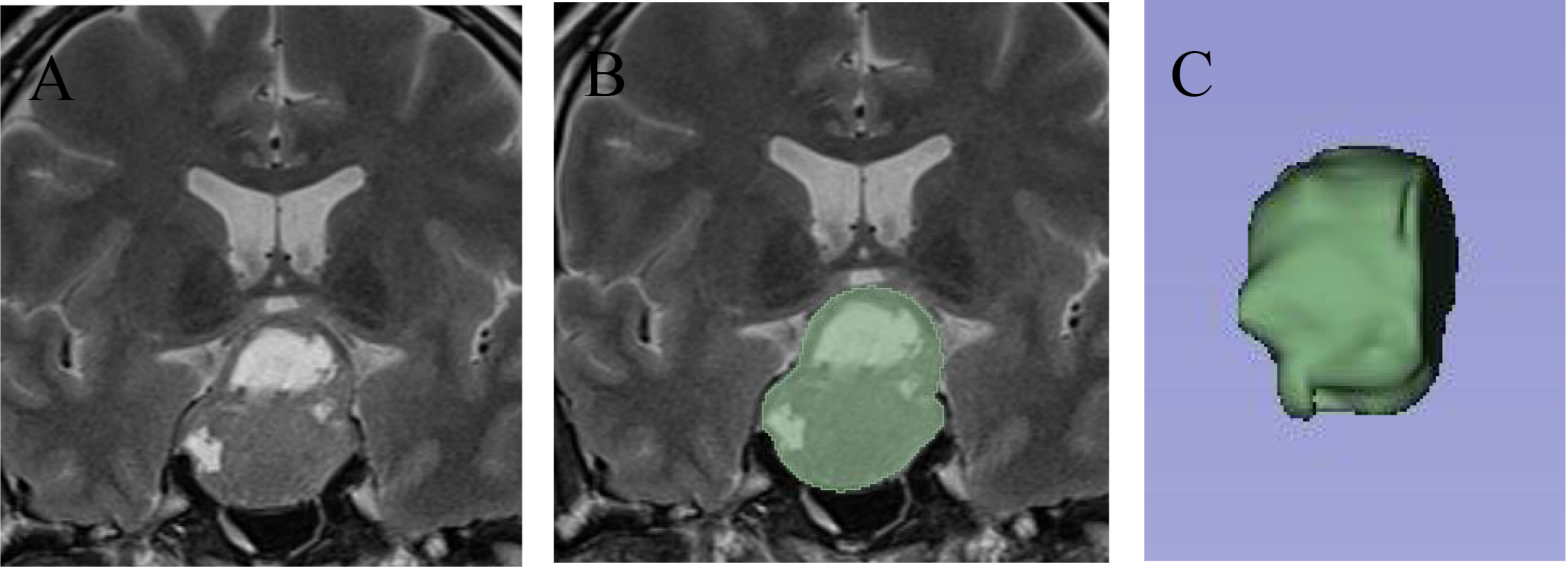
Delineation of the pituitary adenoma ROI using 3D slicer. (A) On T2-weighted imaging, a large pituitary adenoma is seen invading the suprasellar region and cavernous sinus, with cystic changes within the tumor. (B) The 3D Slicer tool was used to outline the full-layer ROI of the pituitary adenoma, showing the complete boundaries of the tumor. (C) After ROI delineation, a three-dimensional reconstruction was performed to visualize the tumor’s spatial structure.
